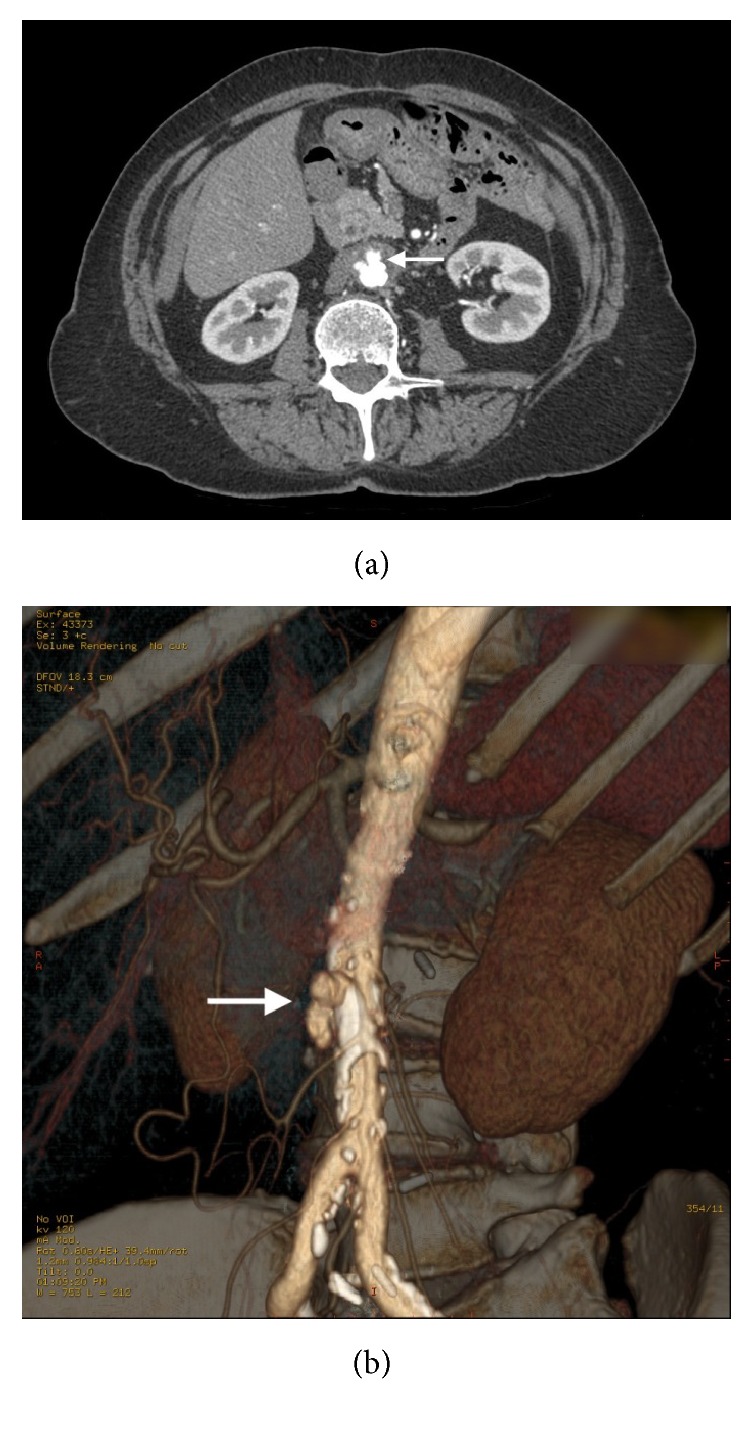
Axial (a) and reconstructed computed tomographic angiography (b) showing signs of prerupture.
